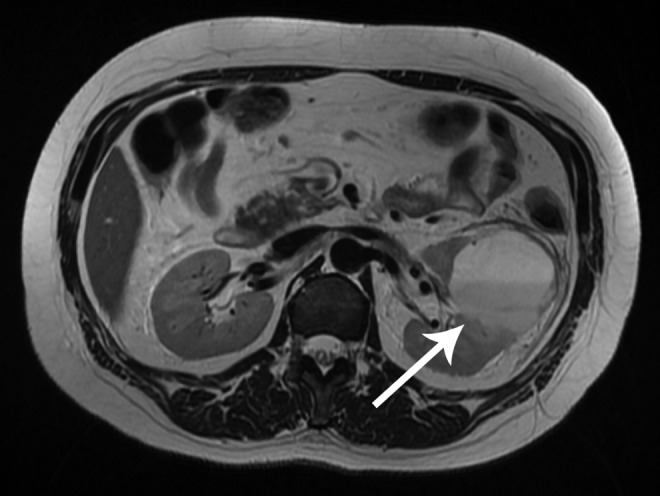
Suspect protrusion of the abscess to the cyst by MRI.
